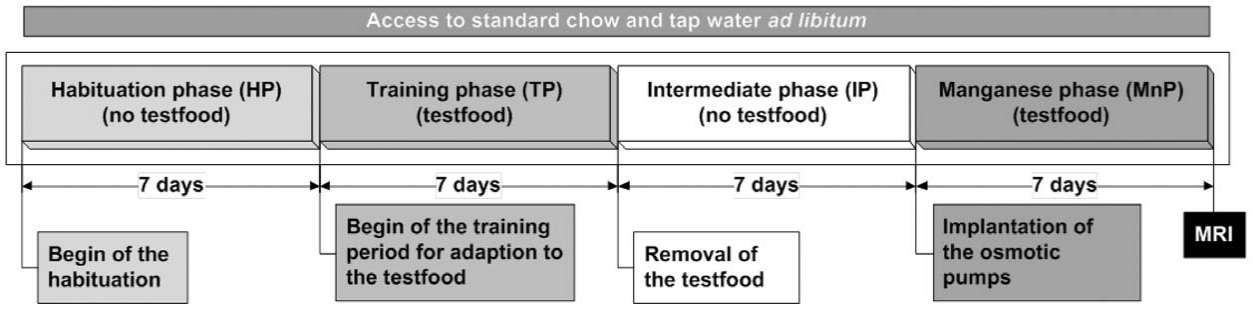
Study design. Overview on the study design for monitoring the influence of food composition on whole brain activity patterns by manganese-enhanced magnetic resonance imaging.
